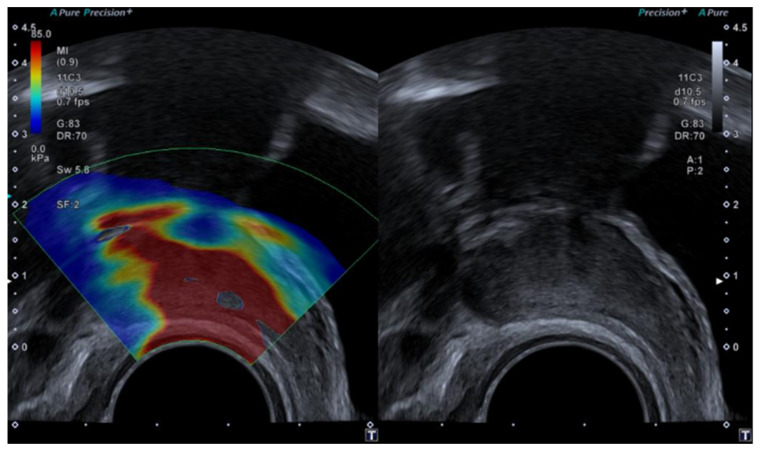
SWE ultrasound showing a t3b tumour in a 70-year-old female.
